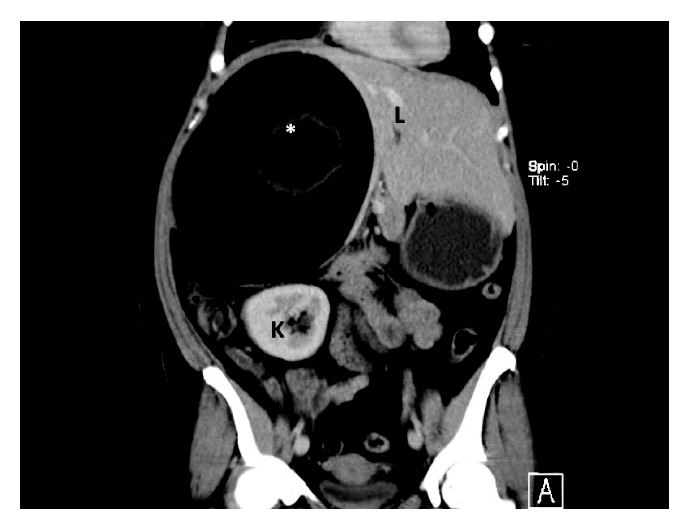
CT scan of the abdomen (coronal view) demonstrating a well-circumscribed cystic mass, with solid components (asterisk), occupying the entire right upper quadrant of the abdomen. The mass measures 19.0 × 19.7 × 13.8 cm in its maximal and displaces the right kidney (K) inferiorly and liver (L) anteromedially.
